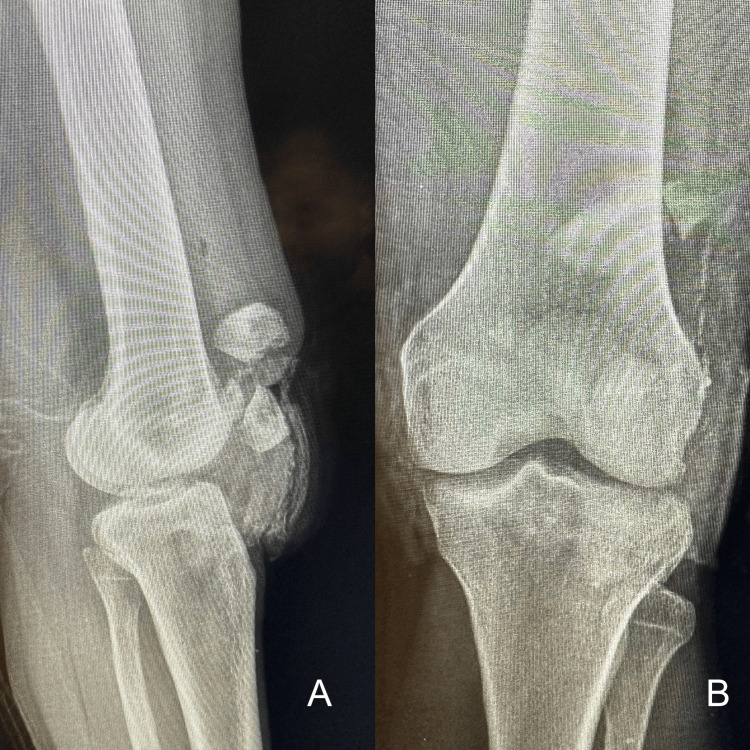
Radiographs obtained on admission to the ED. (A) Anteroposterior view showing a transverse patellar fracture. (B) Lateral view of the knee.
